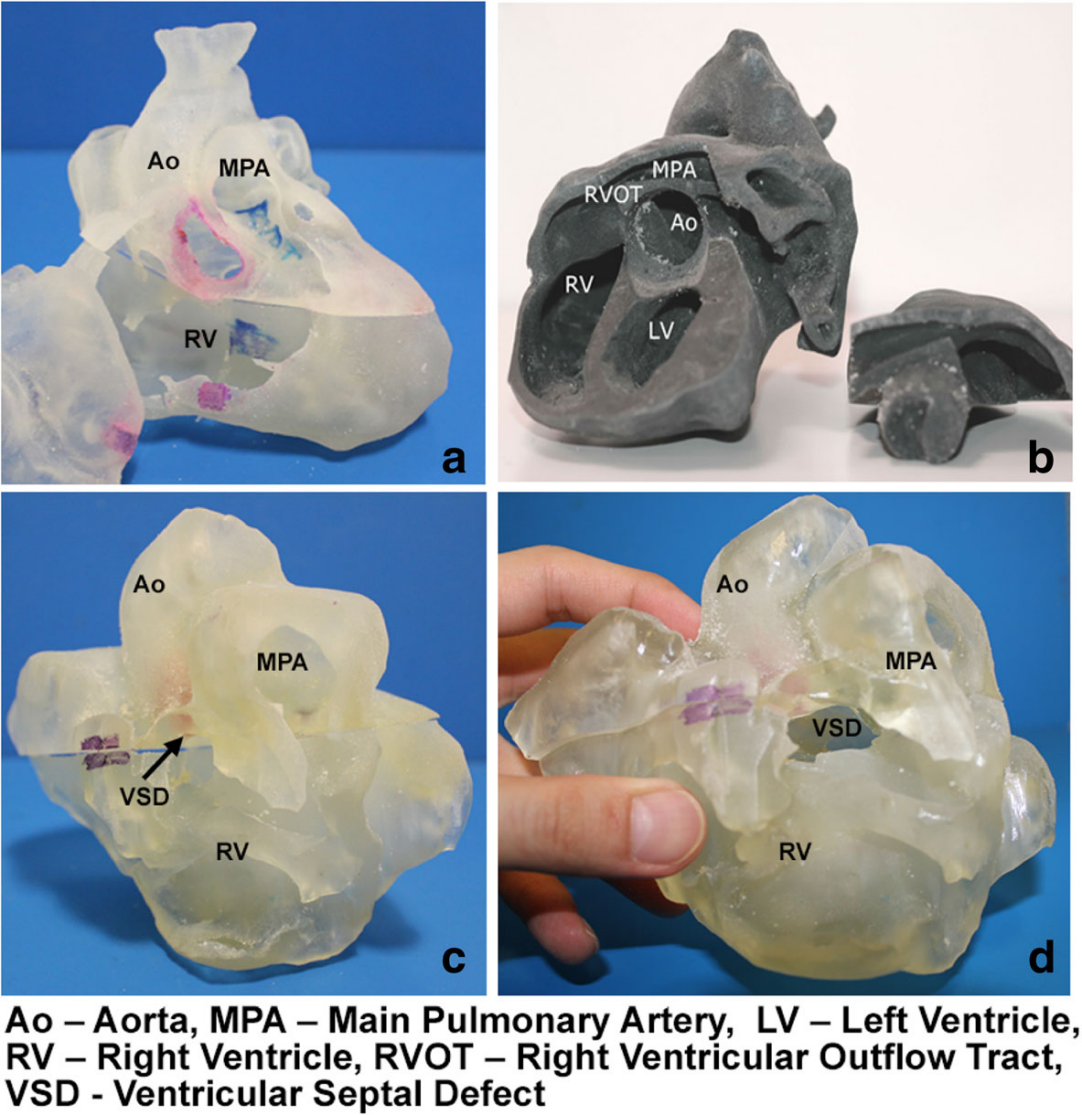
Respective physical models and features are as shown. a Normal heart: This model, created from Cardiac CT, is partitioned into 3 pieces, including an anterior portion (the right ventricular free wall) that can be removed to visualize the normal interventricular septum. The remaining superior and inferior portions can be separated to allow for visualization of the aorta and its position relative to the right ventricle. b Repaired tetralogy of Fallot heart from an adult: The model, created from Cardiac MRI is separated into 2 pieces, well fitted together via “Lego” peg depression. The cut in the main body allows for clear visualization of the pulmonary infundibular stenosis and overriding aorta. c Unrepaired tetralogy of Fallot heart from an infant: The 3D model, created from 3D echocardiogram, was partitioned into 2 pieces; a superior and inferior portion divided along the ventricular septal defect. d Unrepaired tetralogy of Fallot heart from an infant: Separating superior and inferior portions allows for clear visualization of the VSD as well as the aortic override relative to the VSD
